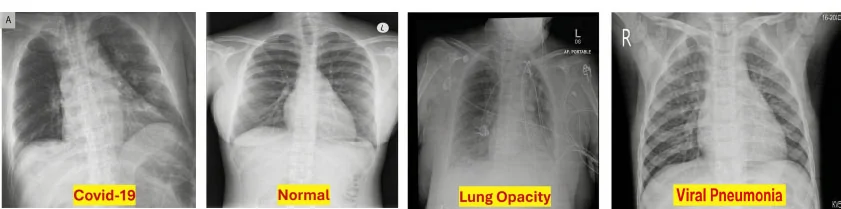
Sample chest X-ray images for different disease categories.
